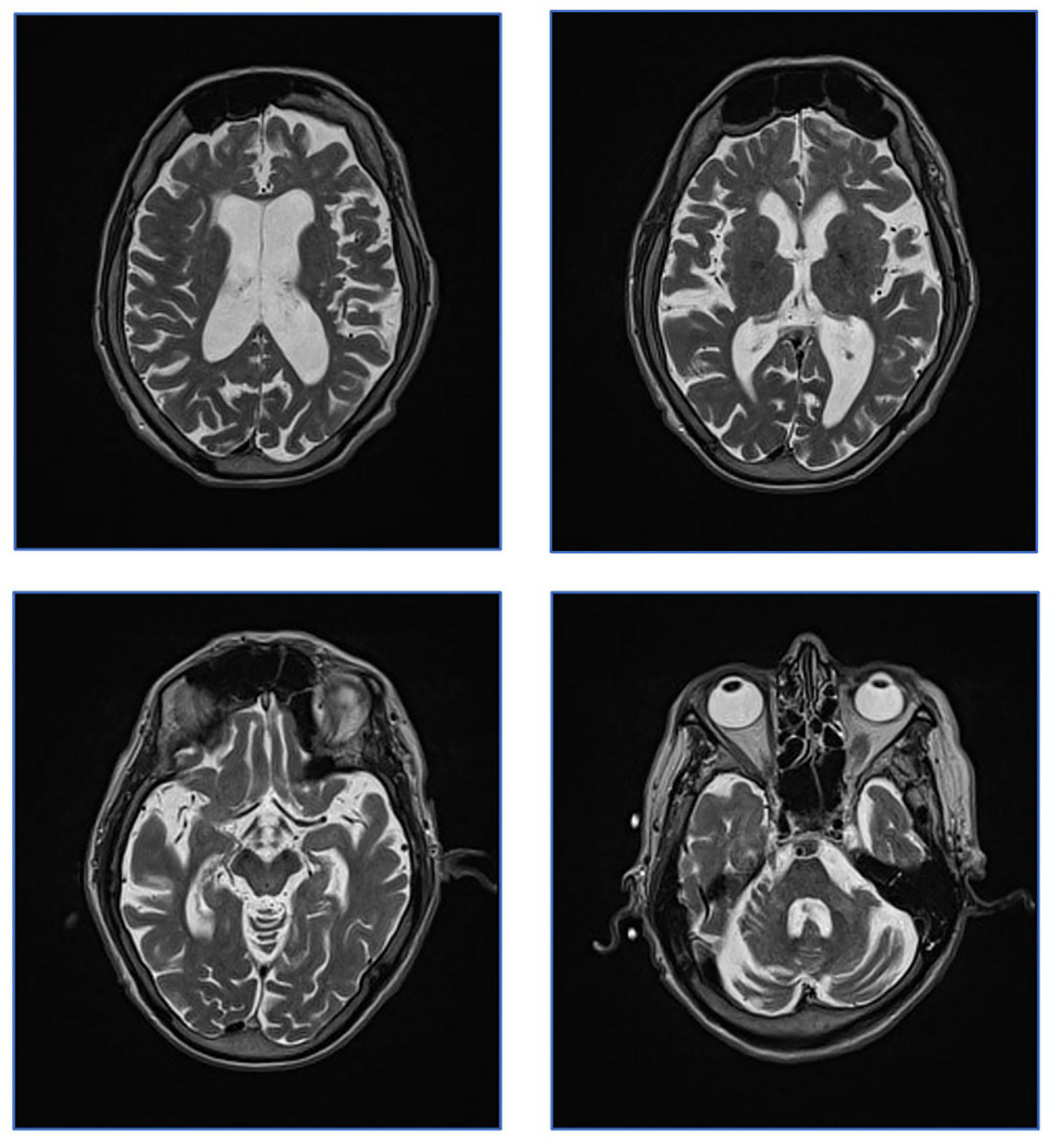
Patient MRI from 2017. Patient MRI. Axial T2 weighed images showing global atrophy and unspecific T2 hyperintensities. Characteristic WD changes to basal ganglia were not present.
